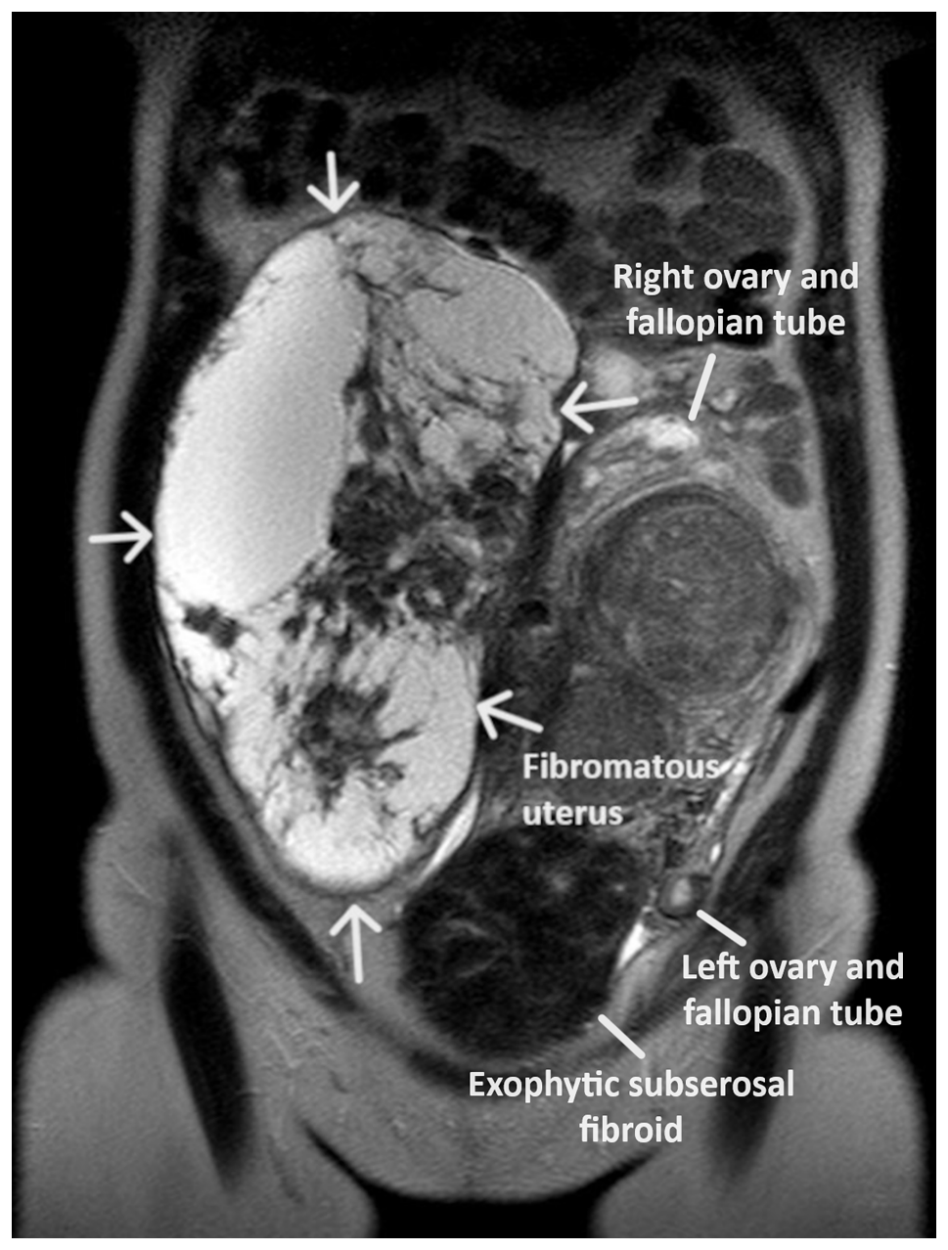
Magnetic resonance imaging of a huge intraligamental leiomyoma of the uterus: The space-occupying lesion is depicted by white arrows with lobulated margins and extensive nodular solid and cystic necrotic elements, nodular wall protrusions and multiple internal septations; it corresponds to a uterine leiomyoma with growth within the broad ligament.
